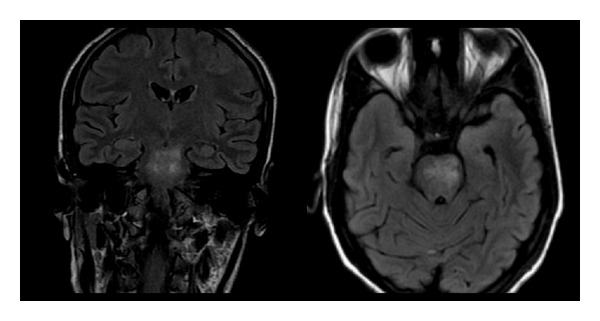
Coronal and axial FLAIR images showing pontomesencephalic hyperintensities suggestive of PRES syndrome.
